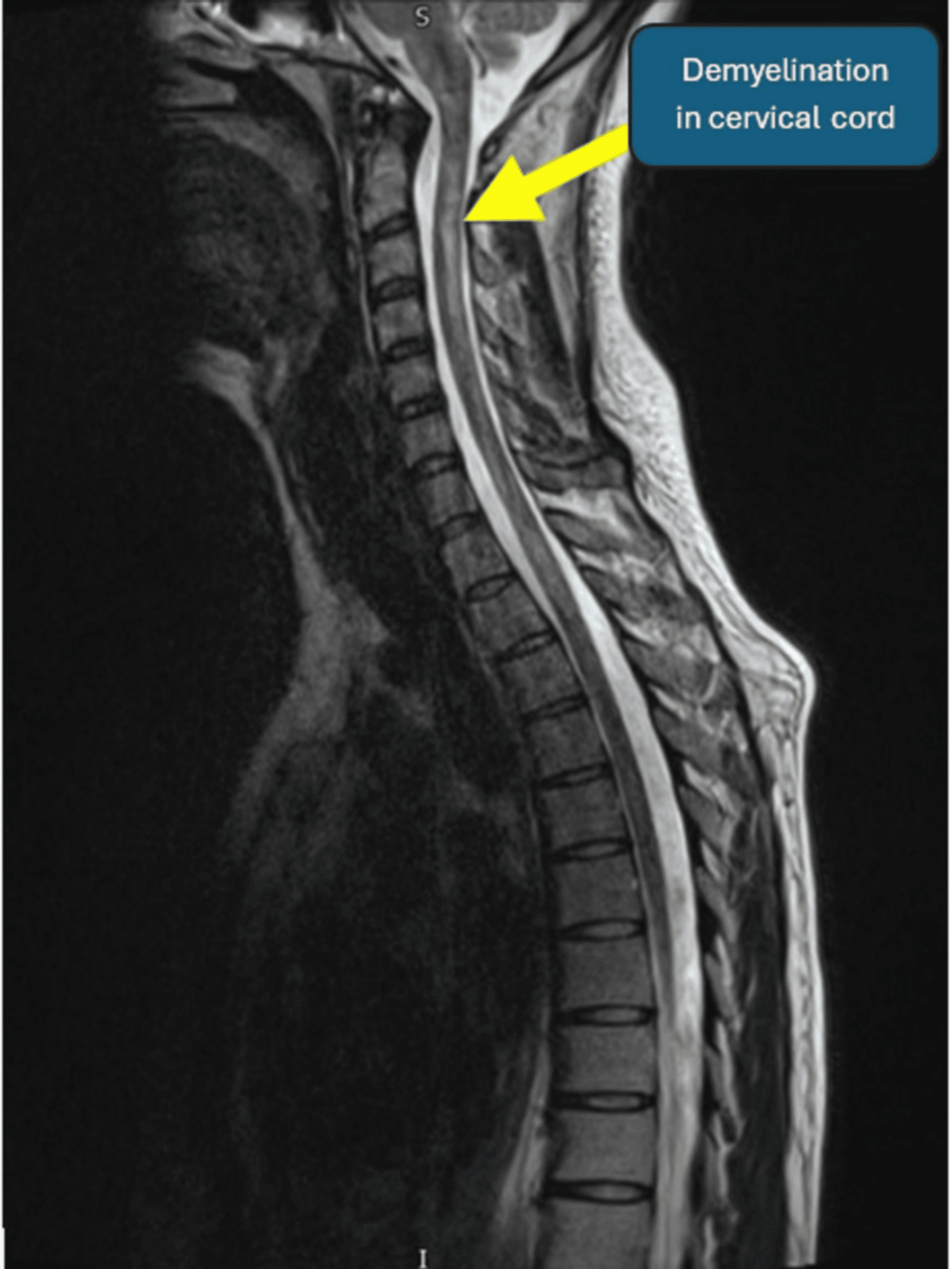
MRI of the spine showing demyelination in the cervical spine
